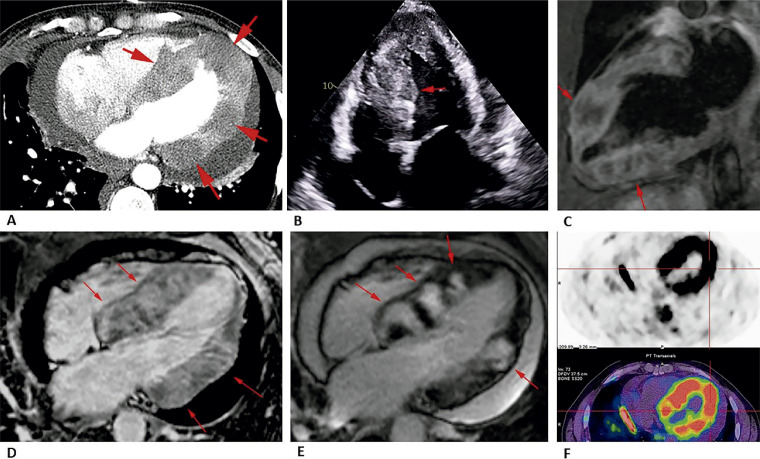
Diferentes modalidades de imagem evidenciando múltiplas metástases intracardíacas. A) TC de tórax; B) Ecocardiografia transtorácica; CE) RM cardíaca mostrando lesões hipointensas nas imagens ponderadas em T2 com halo periférico de hiperintensidade (C); realce heterogêneo precoce (D) e realce tardio intenso com gadolínio (E); F) captação anormal de fluorodesoxiglicose no 18F-FDG PET/CT.
